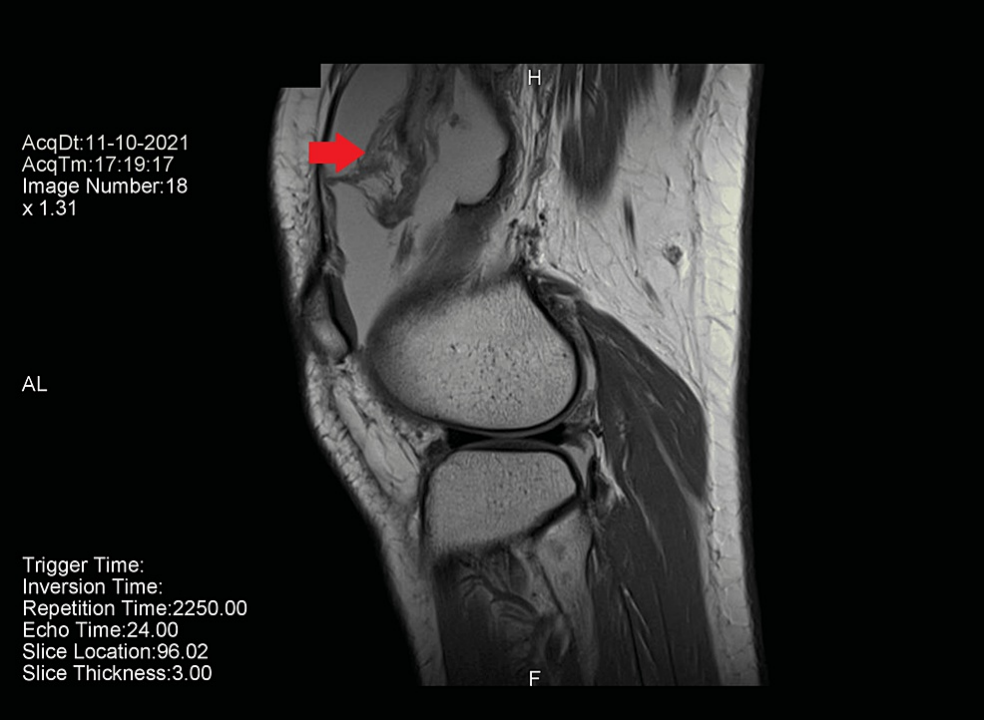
MRI of the knee joint in a longitudinal section (arrow points to the tumor). The tumor is surrounded by an effusion.
